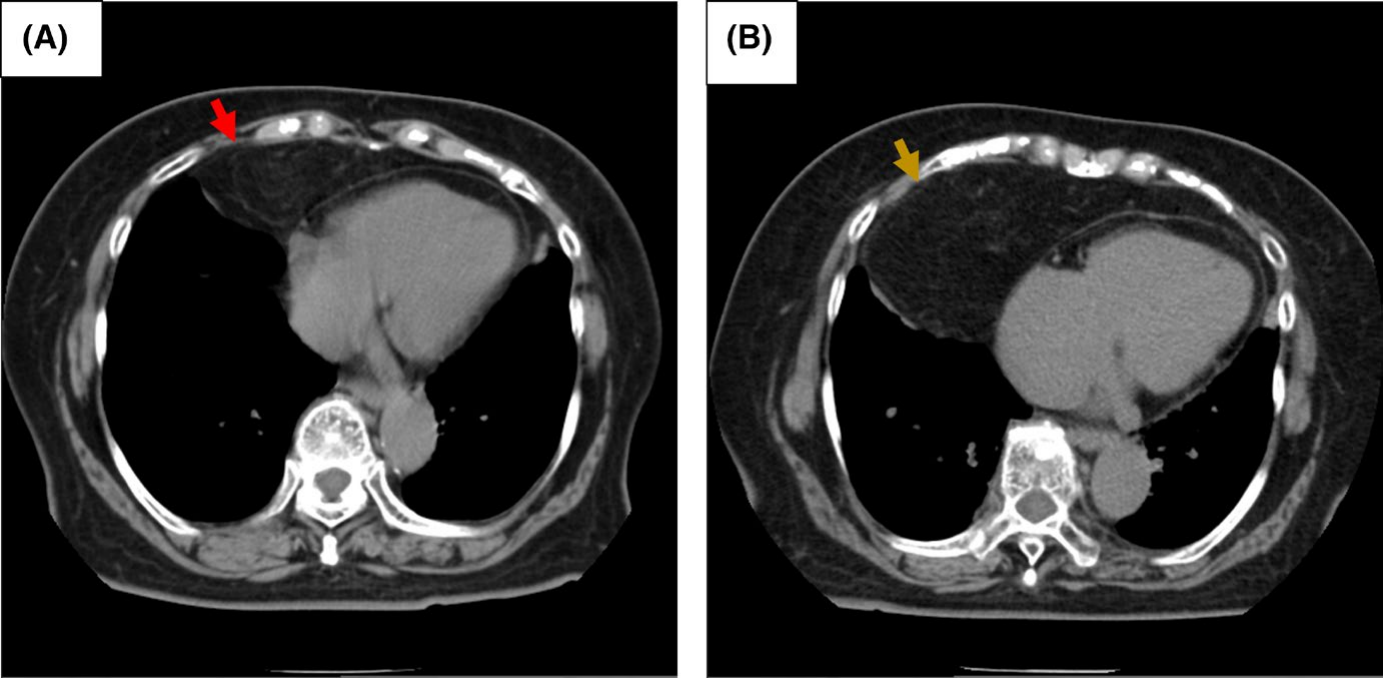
Findings of the thoracic CT without enhancement. (A) on her first visit, (B) nine years later. Thoracic CT showed a homogenous mass shadow measuring 17.4 cm × 4.5 cm × 6 cm in her right anterior mediastinum (red arrow). The mass shadow has significantly increased in the size of the mass to 18.9 cm × 8.0 cm × 8.0 cm in the past 9 years (yellow arrow)
